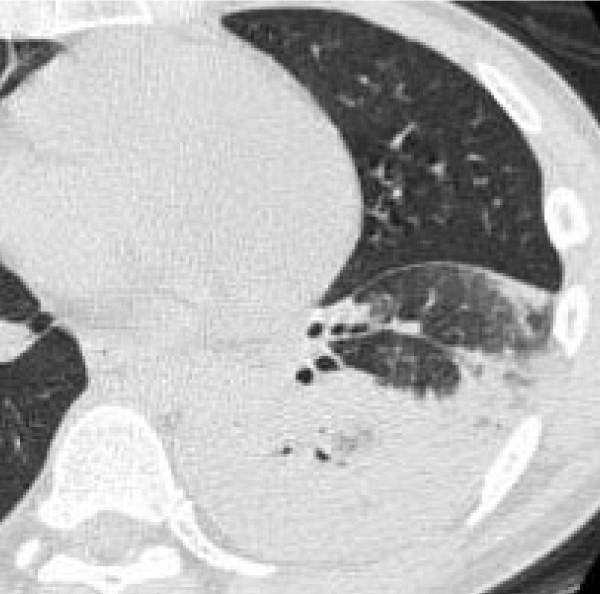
39-year-old man with *S. pneumoniae *pneumonia. CT shows air-space consolidation in left lower lobe.
